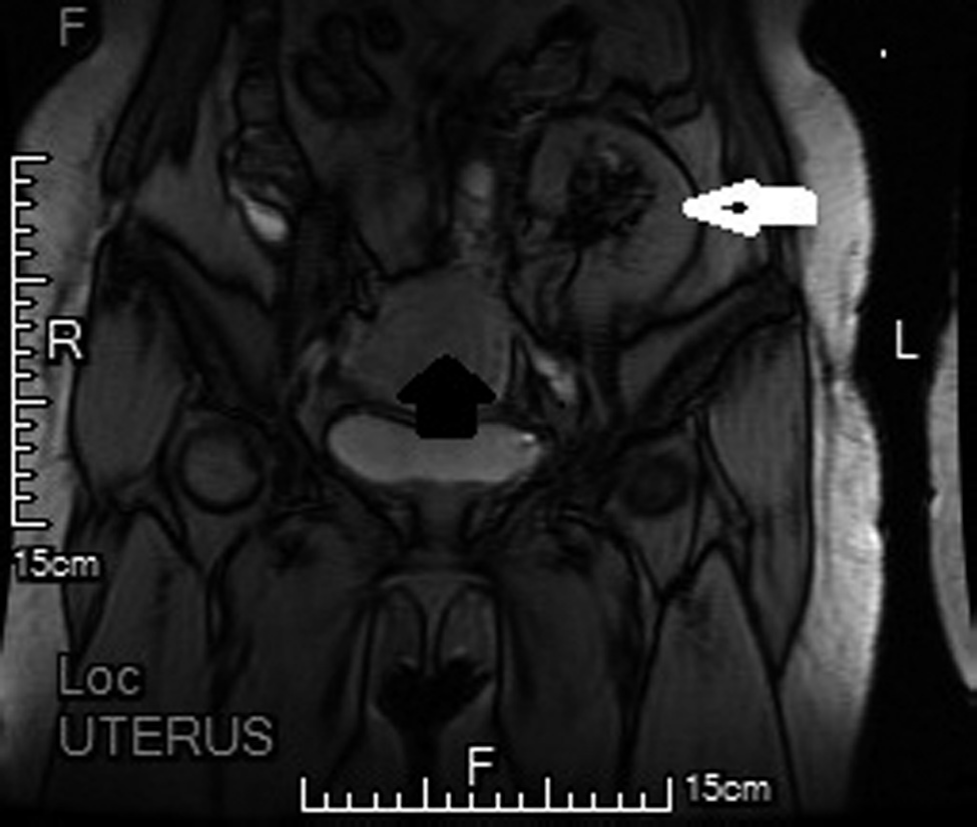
Preoperative MRI image. The white arrow indicates the transplanted kidney. The black arrow indicates the FIGO-stage IA endometrial carcinoma.
